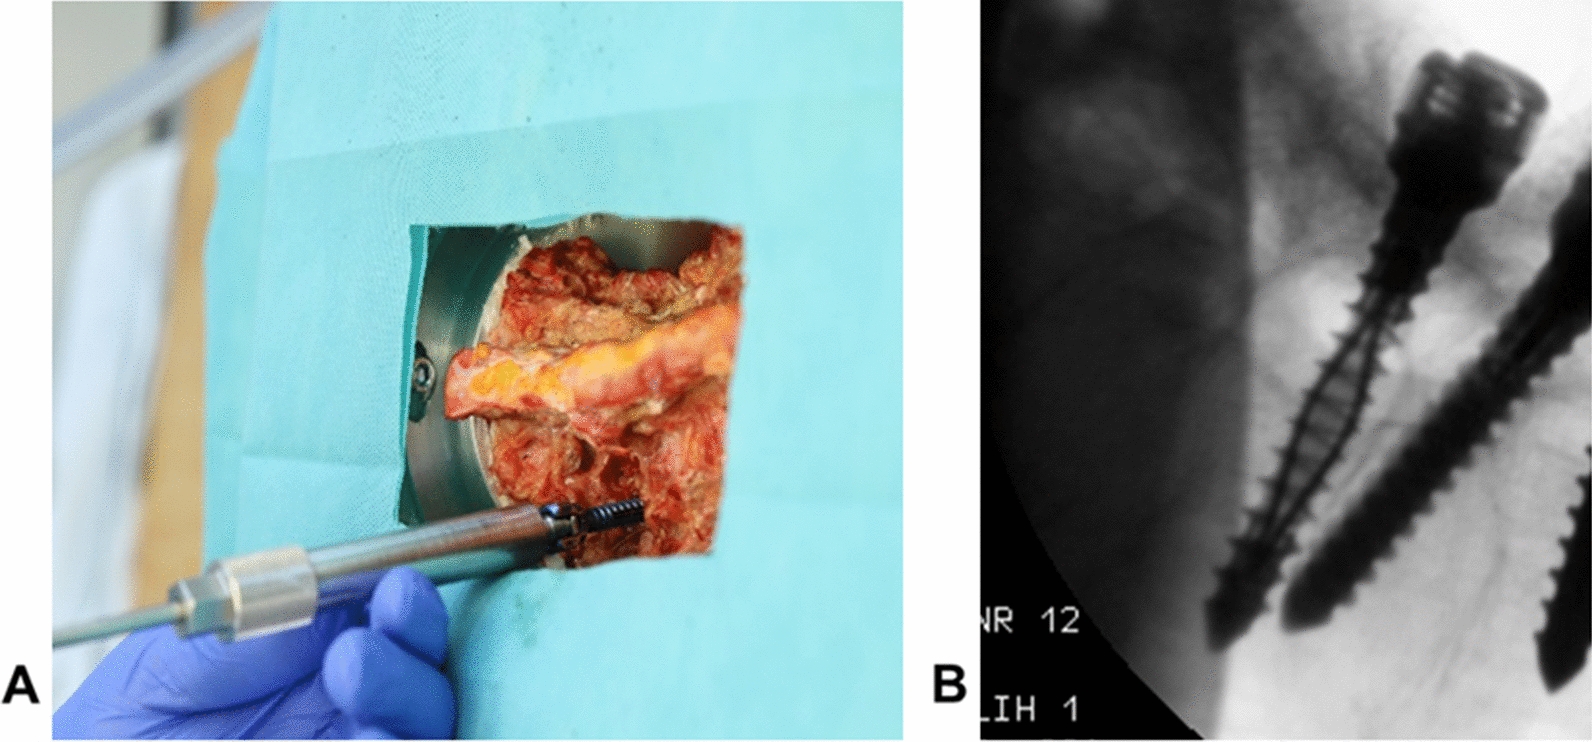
Handling test. A Implantation of the NiTi screw; B CT image of the implanted NiTi screw
